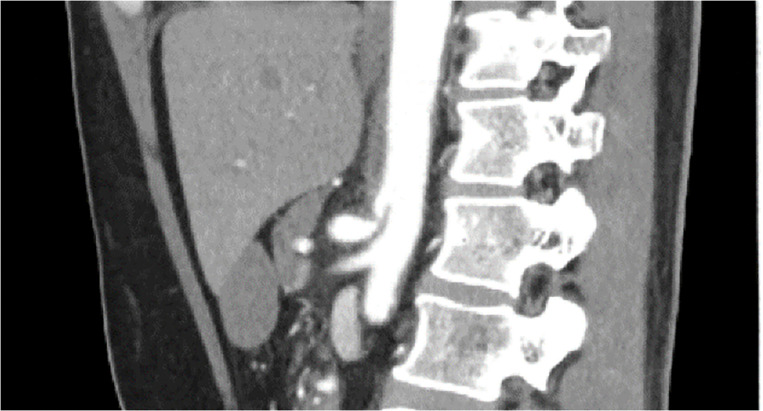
Contrast-enhanced CT on initial admission showing the primary pancreatic lesion.
